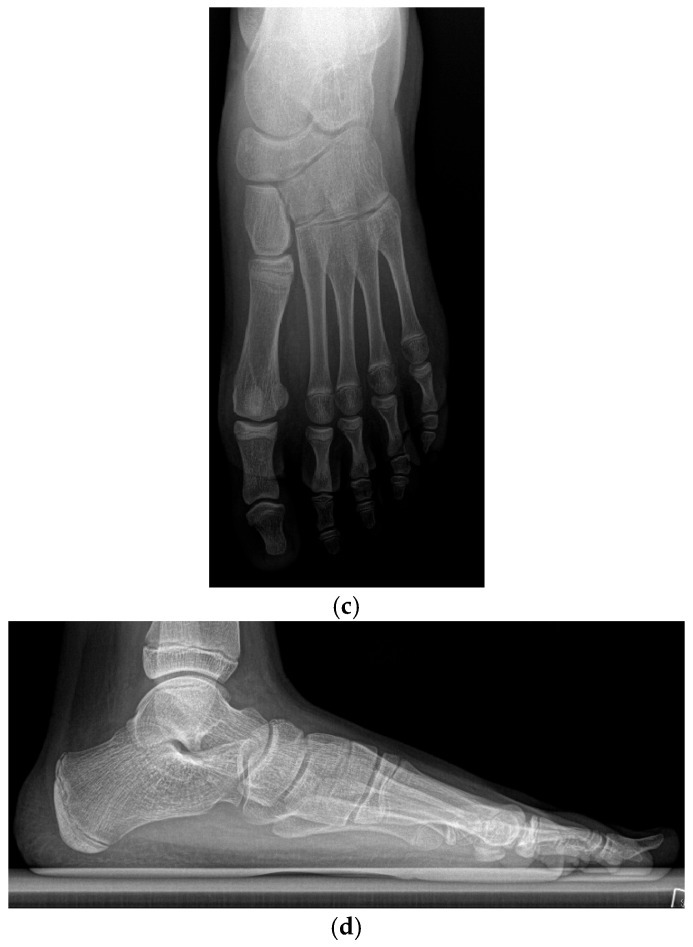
(a,b) Antero-posterior and lateral standing radiographs of the left asymptomatic flatfoot of a 7-year-old boy. The talo-navicular coverage angle measured 42°, while Meary’s and pitch angles measured, respectively, −14° and 13°. Less-invasive surgery was planned, but it was never performed. (c,d) The same foot when the boy was 14 years old. The medial arch of the foot self-recovered without any treatment, as shown by the talo-navicular coverage angle, Meary’s and pitch angles that measured respectively 29°, 0° and 20°.
